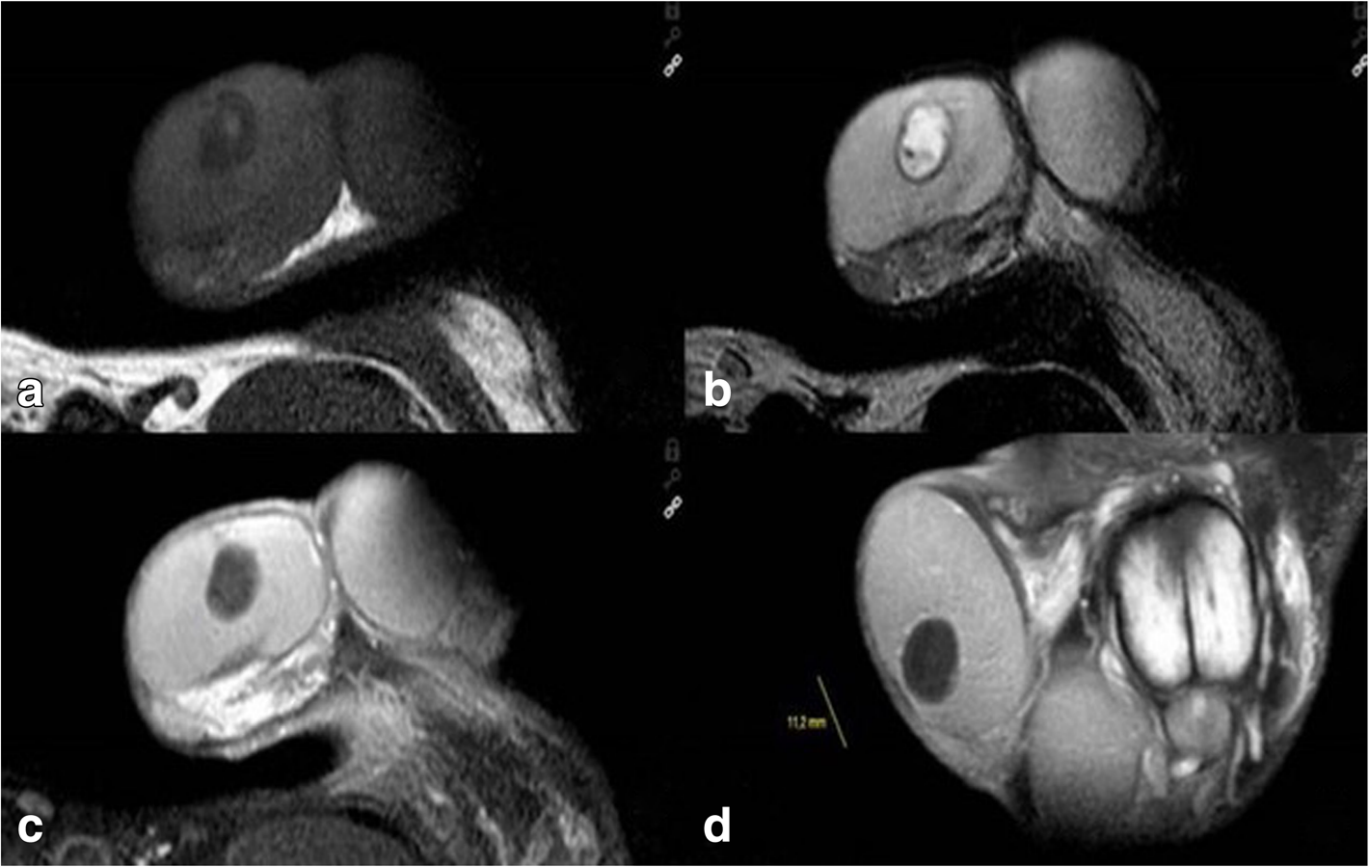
Scrotal MRI of patient with simple testicular epidermoid cyst, 1.5 Tesla MRI, surface coil. a T1 weighted imaging showing cyst with lower signal intensity than testicular parenchyma. b. T2-weighted imaging: typical bull-eye appearance of epidermoid cyst. Note the high signal intensity within cyst core. c and d. T1-weighted imaging with application of gadolinium-based contrast material. Note signal enhancement in testicular parenchyma but not in the cyst core highlighting the avascular area within the cyst
